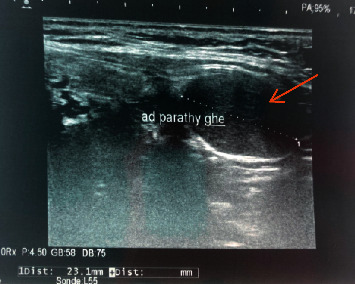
Cervical ultrasound showing an oval, well-defined, hypoechoic nodule, with regular contours, measuring 33 ∗ 32 ∗ 18 mm (red arrow).
